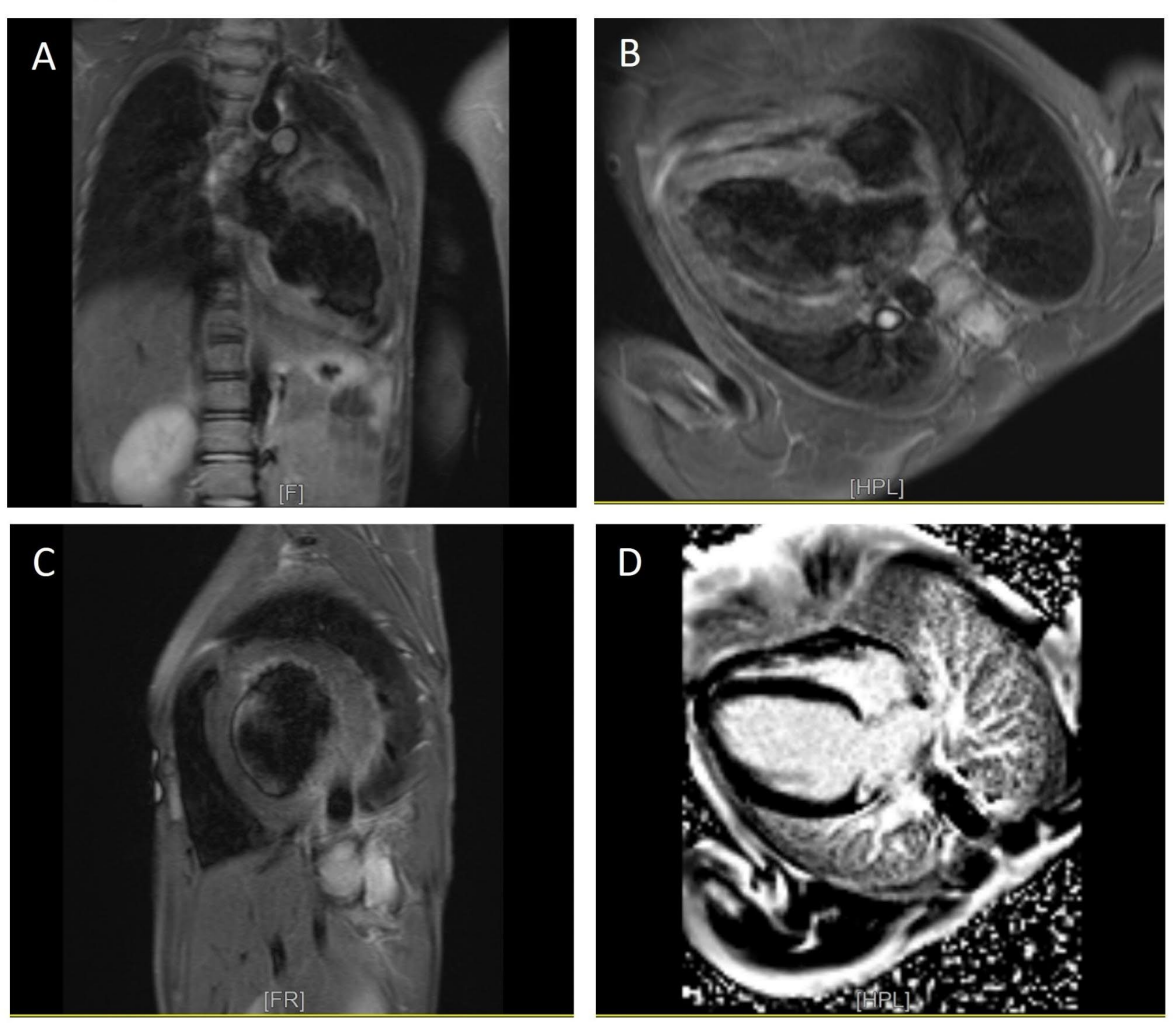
The image shows the proband’s cardiac magnetic resonance imaging. A, B, and C: They present 2- and 4-chamber views and short-axis short tau inversion recovery (STIR) images, respectively. Severe left ventricular enlargement is observed. D: The 4-chamber phase-sensitive inversion recovery (PSIR) with a 10-minute delay demonstrates no abnormal enhancement. Increased signal intensity adjacent to the subendocardial muscles in the STIR images is secondary to a significantly reduced ejection fraction and subsequent blood stasis
